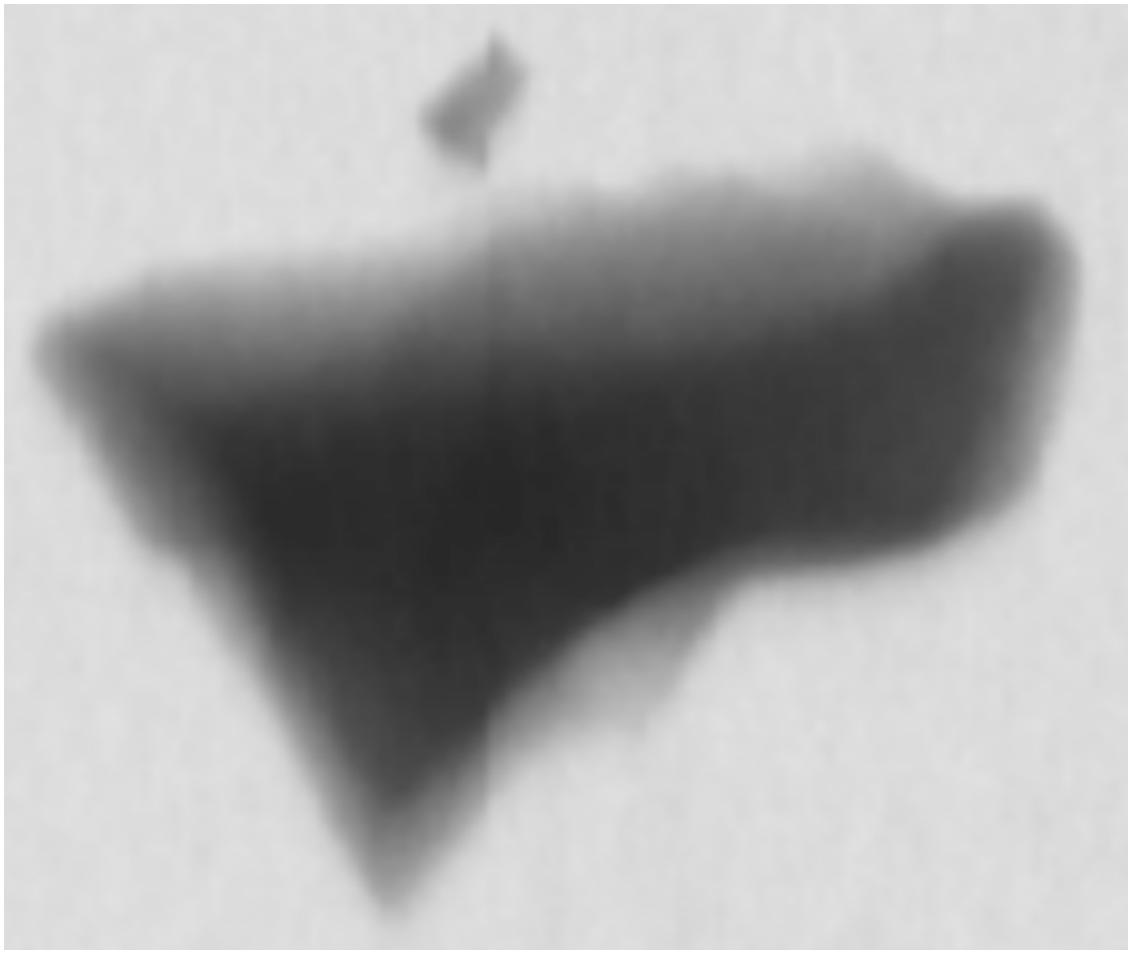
Gray image obtained from X-ray detector.
